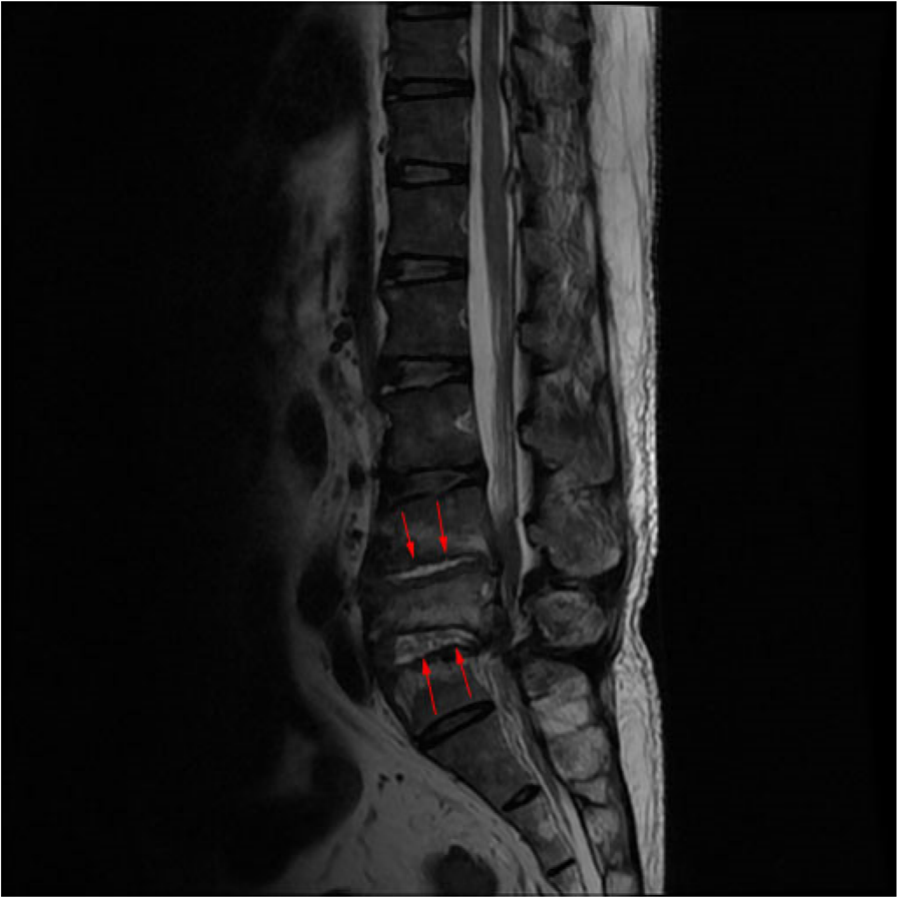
Hyperintensity within the intervertebral discs spaces of L4/L5 and L5/S1 on T2-weighted imaging in keeping with fluid within. Fluid within the L4/L5 and L5/S1 intervertebral discs spaces (*arrows*) on T2-weighted imaging
